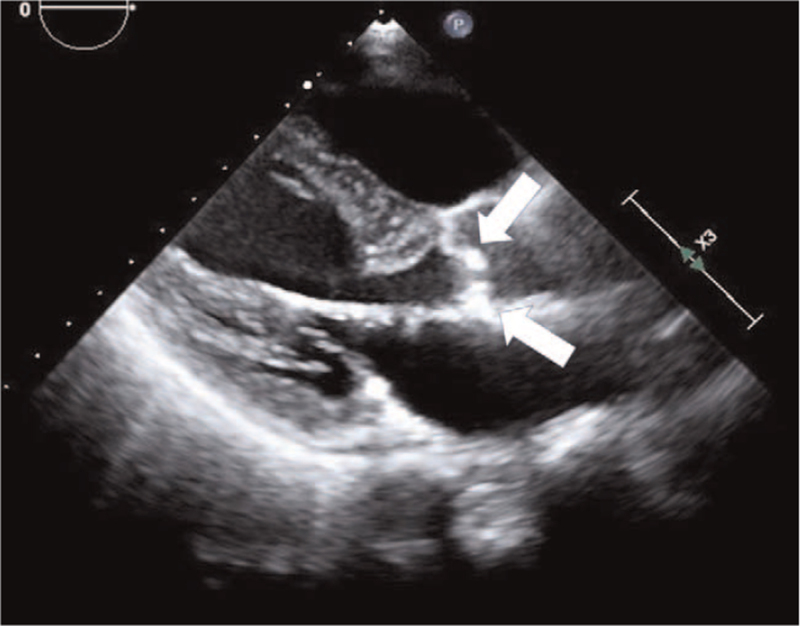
Transthoracic echocardiography findings. Transthoracic echocardiography long-axis view of the 4 cavities shows severe calcification of the aortic valve and restricted aortic valve leaflet motion during systole (white arrows).
